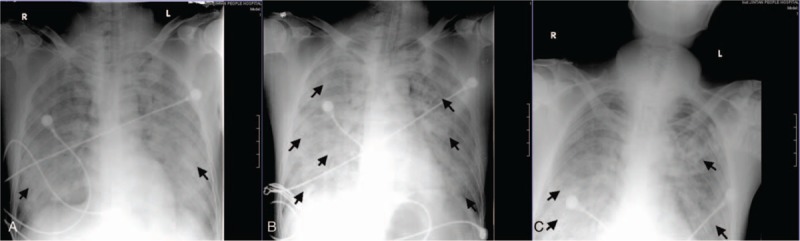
Serial CXRs taken at 1 (A), 2 (B), and 5 (C) days of admission. Arrow heads show interstitial and alveolar pulmonary edema. CXR = chest x-ray.
